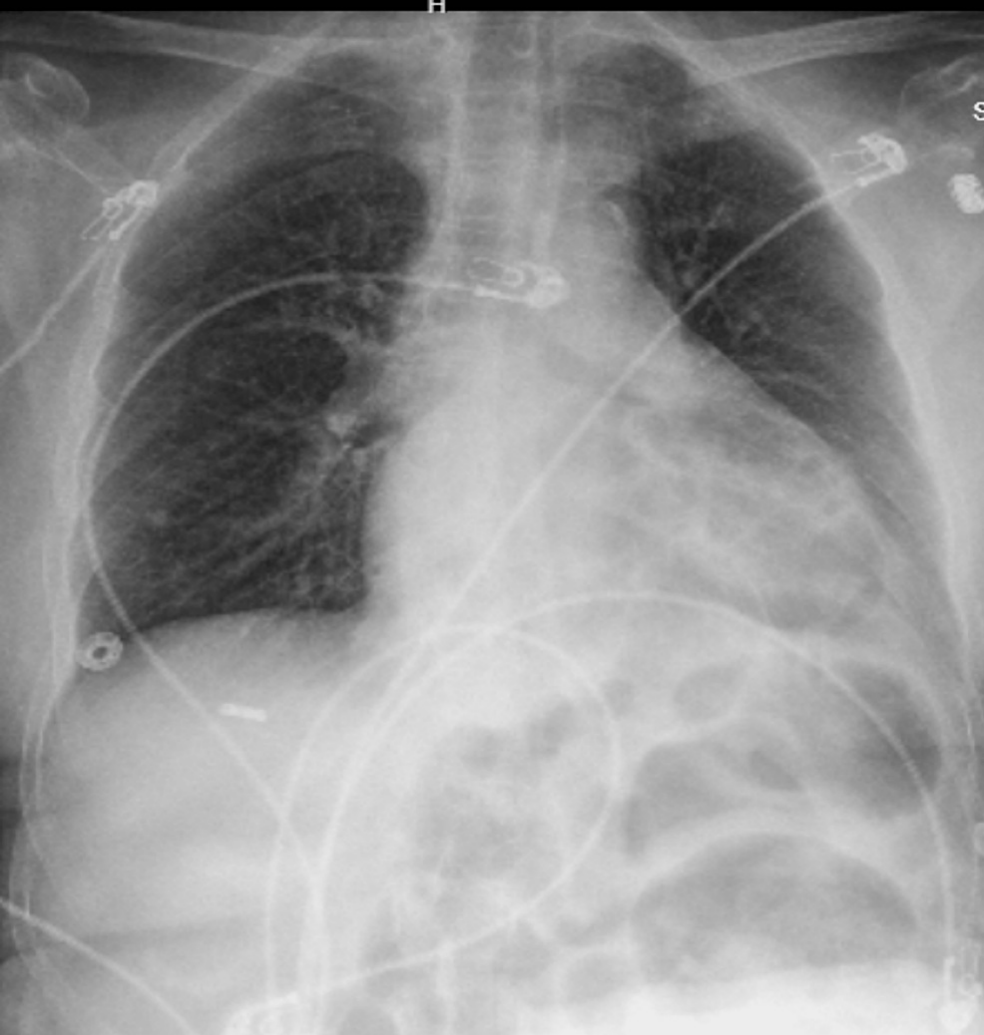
Chest x-ray depicting bowel in the thoracic cavity
